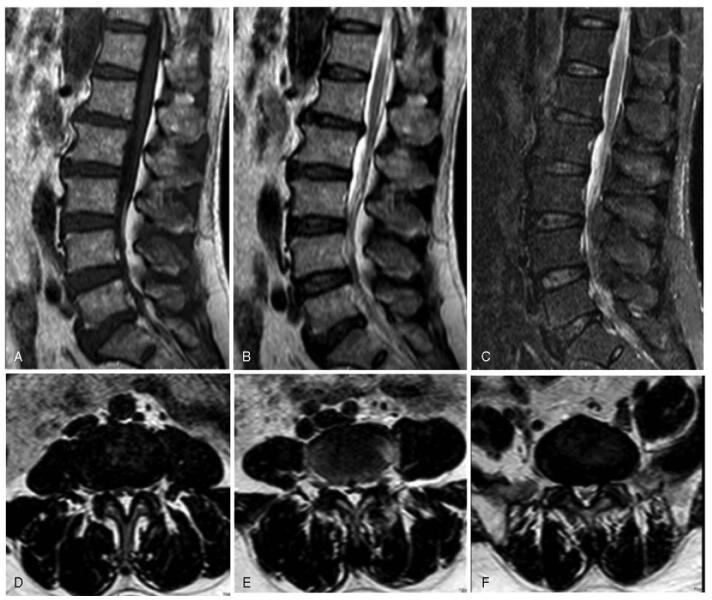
Lumbar MRI showed that the adipose tissue compresses the thecal sac at L3–S1 levels. (A) T1-weighted sagittal image, (B) T2-weighted sagittal image, (C) fat-suppressed T2-weighted image, (D) lumbar axial MRI (L3–L4 level), (E) lumbar axial MRI (L4–L5 level), and (F) lumbar axial MRI (L5–S1 level). MRI = magnetic resonance imaging.
